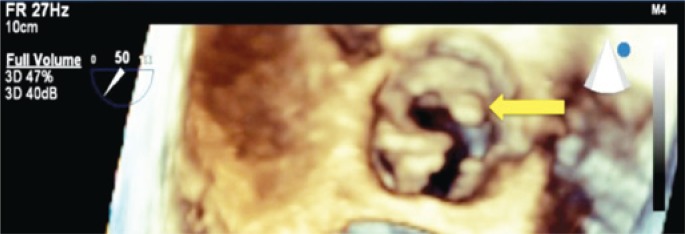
Three-dimensional transthoracic echocardiogram showing aortic leaflets thickness-systole (arrow)
